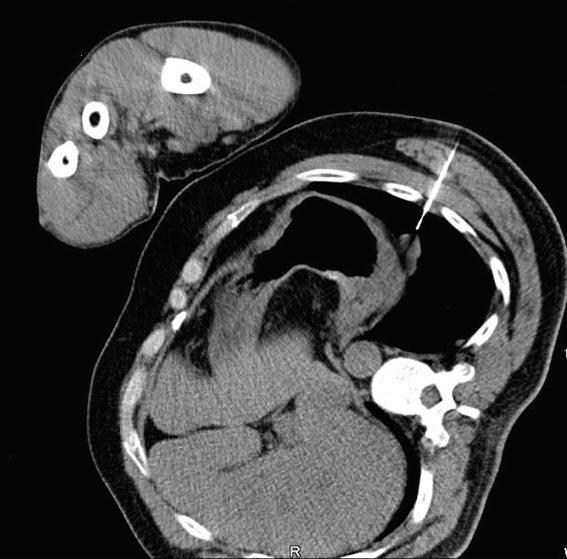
CT-guided biopsy of the mass which subsequently ruled out malignancy.
